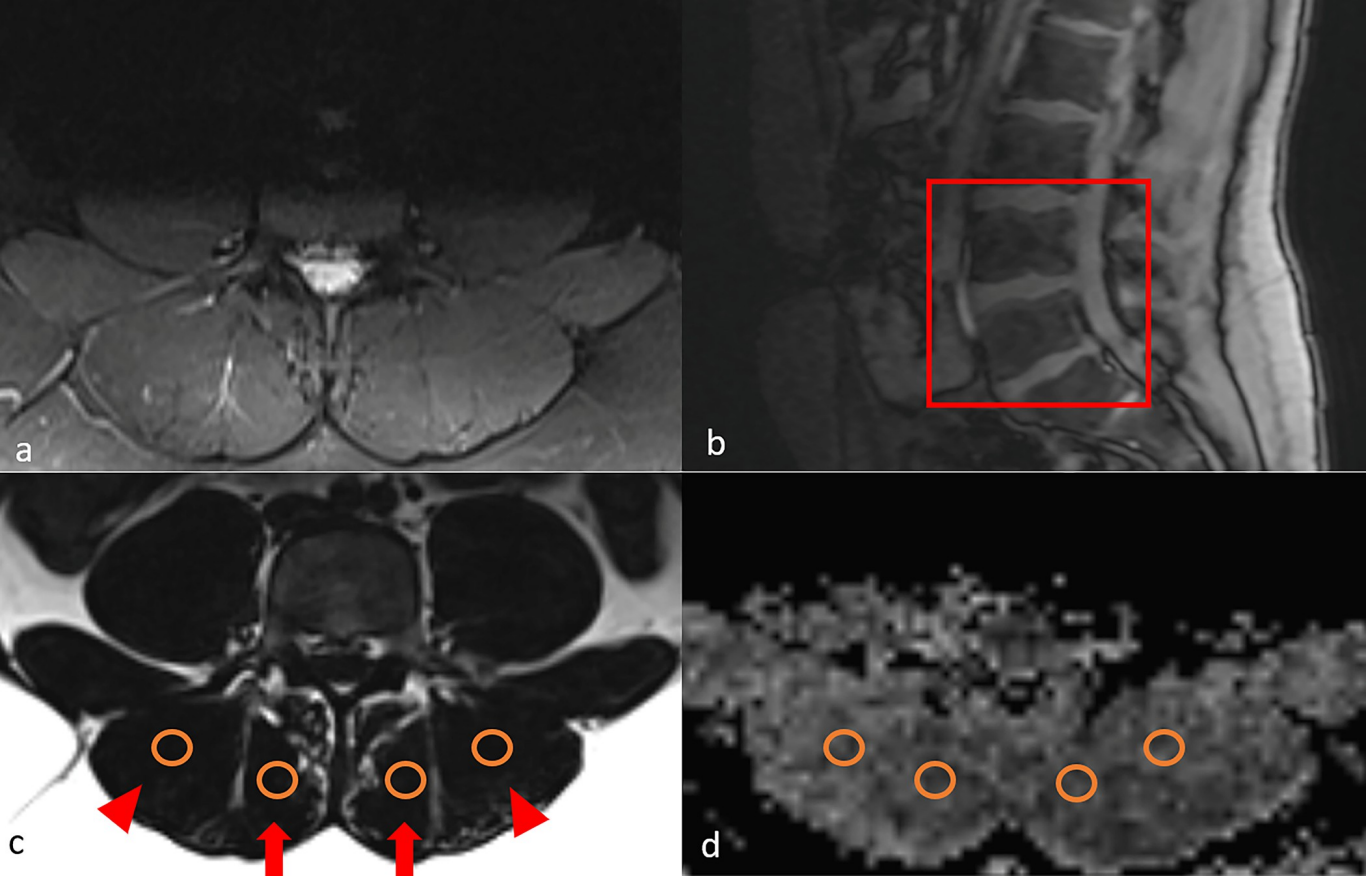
Magnetic resonance imaging analysis. Qualitative image analyses were performed on fat-suppressed T2-weighted images (a) and T1 Dixon images (c). Regions of interest (ROIs) were seeded from the upper vertebral endplate level of the L4 vertebra to the lower endplate level of the L5 vertebra (b). Exemplary ROI seeding on fat-only T1 Dixon images in the erector spinae muscle (arrowheads) and multifidus muscle (arrows) (c). ROIs were then transferred to the corresponding tensor maps (d).
